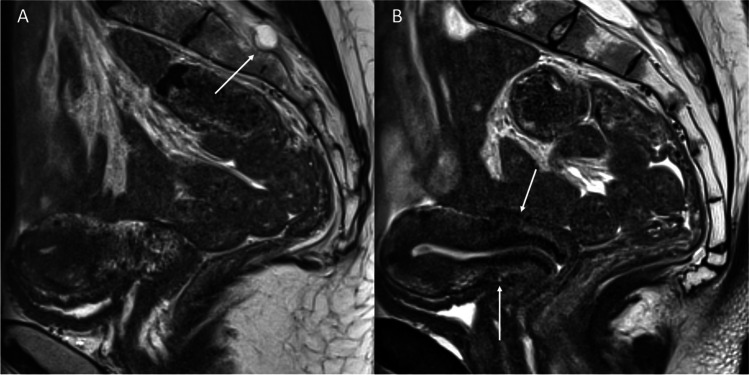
T2-weighted MR images of the pelvis in a 29-year-old female with a Tarlov cyst referred for assessment of pelvic pain. A dedicated pelvic MR was obtained in view of a history of cyclical pain. A Sagittal image shows the Tarlov cyst associated with the right S3 nerve. B Sagittal aligned-with-cervix image shows T2-hypointense plaques along the dorsal uterine serosal surface and vesicouterine recess (arrows) which are consistent with endometriosis
